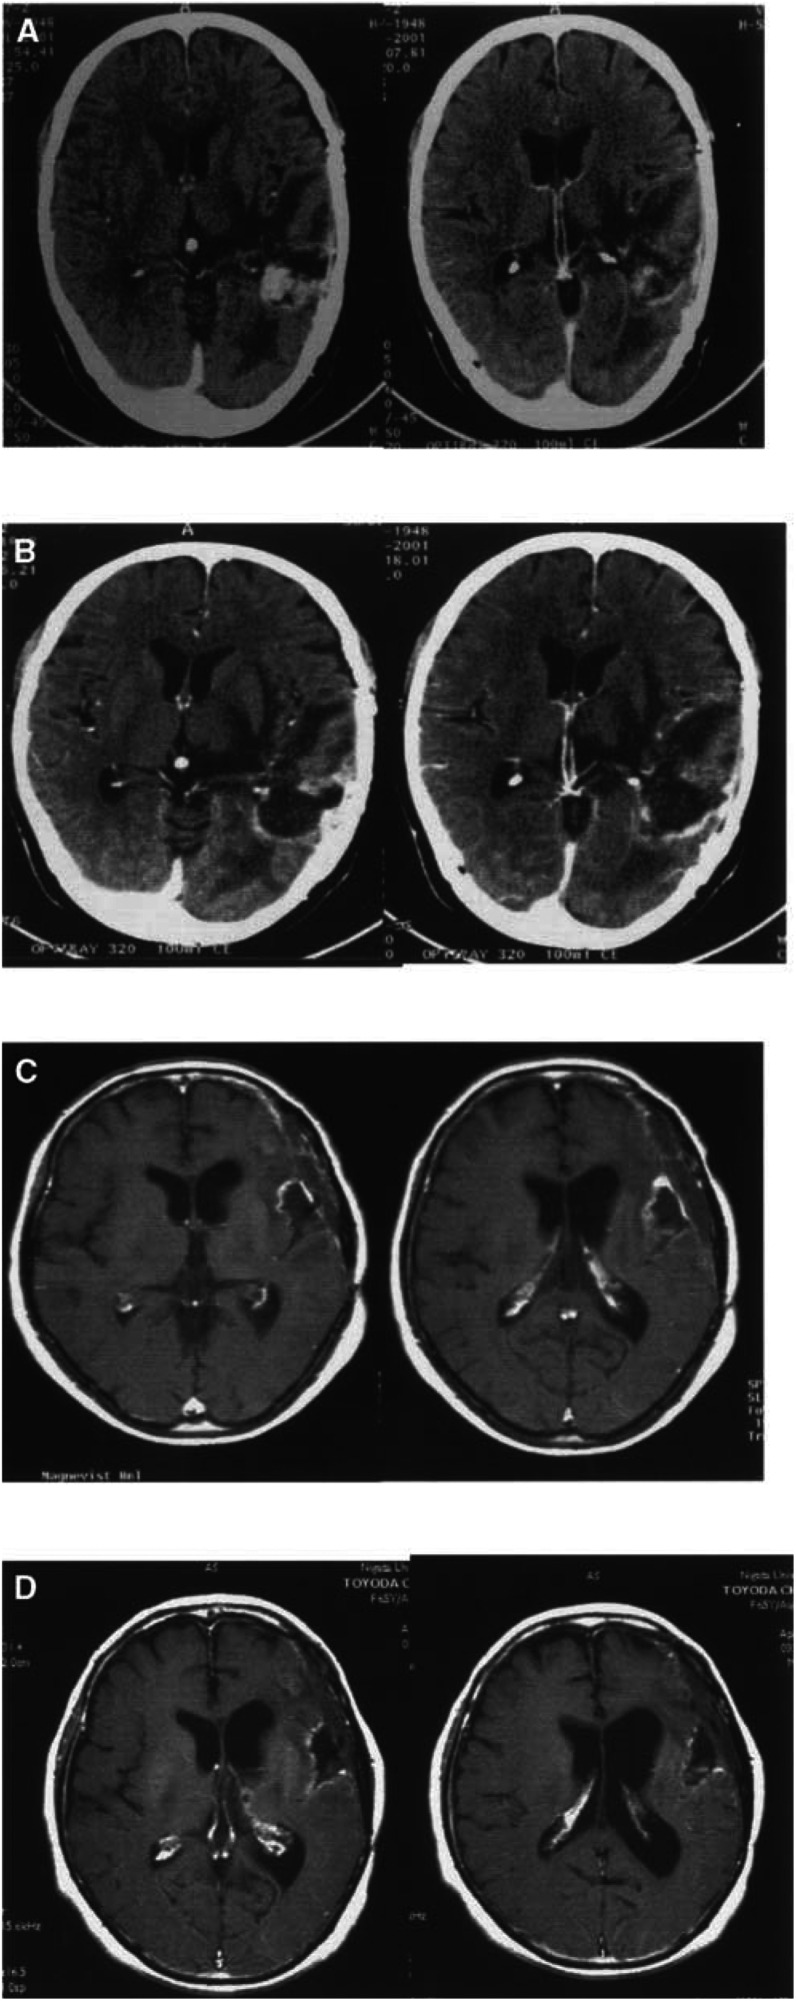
Computed tomography of Case 3 shows that, although the size of the low density area did not change, the contrast enhanced lesion was decreased. Computed tomography contrast enhanced images before (A) and after (B) vaccination. Magnetic resonance imaging of Case 6 shows that the contrast enhanced lesion was decreased after vaccination. Contrast enhanced MRI before (C) and after (D) vaccination.
